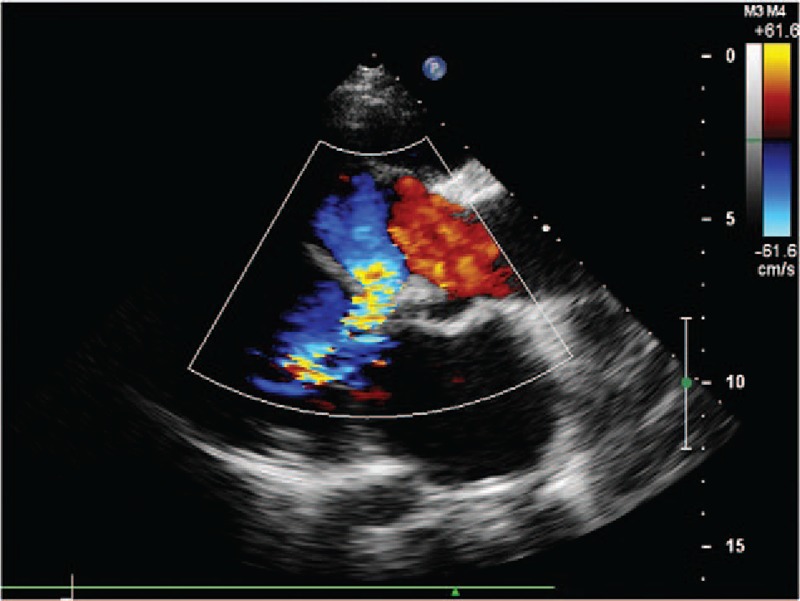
(Patient #7) Transthoracic echocardiogram (parasternal long axis view with color) showing both the communication between the right sinus of Valsalva aneurysm and the dissecting cavity in the interventricular septum and the communication between the dissecting cavity in the interventricular septum and the left ventricle (see also video 2).
